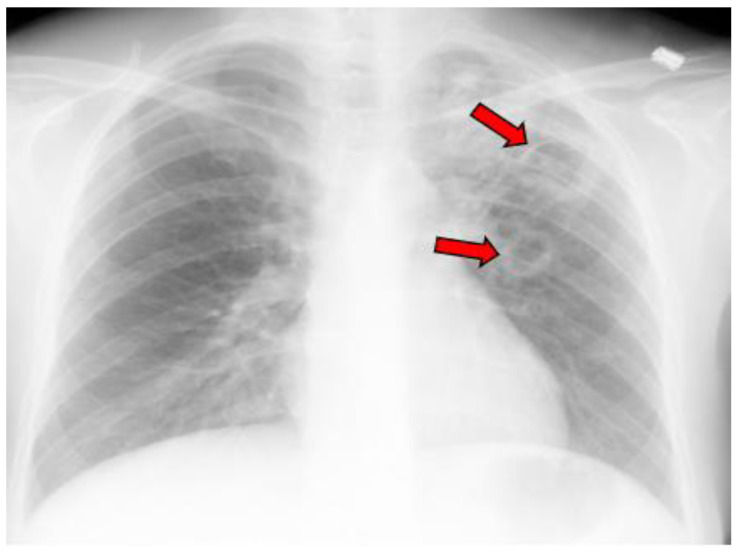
X-ray image of multiple coccidioidal pulmonary cavities. The red arrows indicate cavities.
